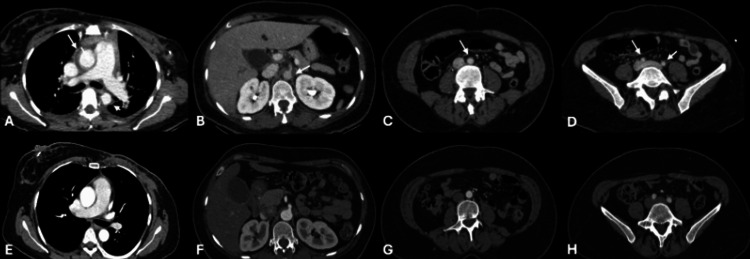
Contrast-enhanced computed tomography (venous phase) of the aortic axis before and after treatment. Upper row (A–D): Axial images from the pre-treatment study demonstrating laminar, concentric, hypodense, non-stenotic thickening of the aortic wall, consistent with periaortitis, involving the ascending and descending thoracic aorta (white arrows) (A), the suprarenal abdominal aorta with extension to the origin of the celiac trunk (B), the infrarenal abdominal aorta (C), and the common iliac arteries (D). Lower row (E–H): Axial images from the post-treatment study, obtained at the same anatomical levels, showing complete resolution of the wall thickening, with preserved aortic caliber and morphology, and no residual signs of vascular inflammation.
